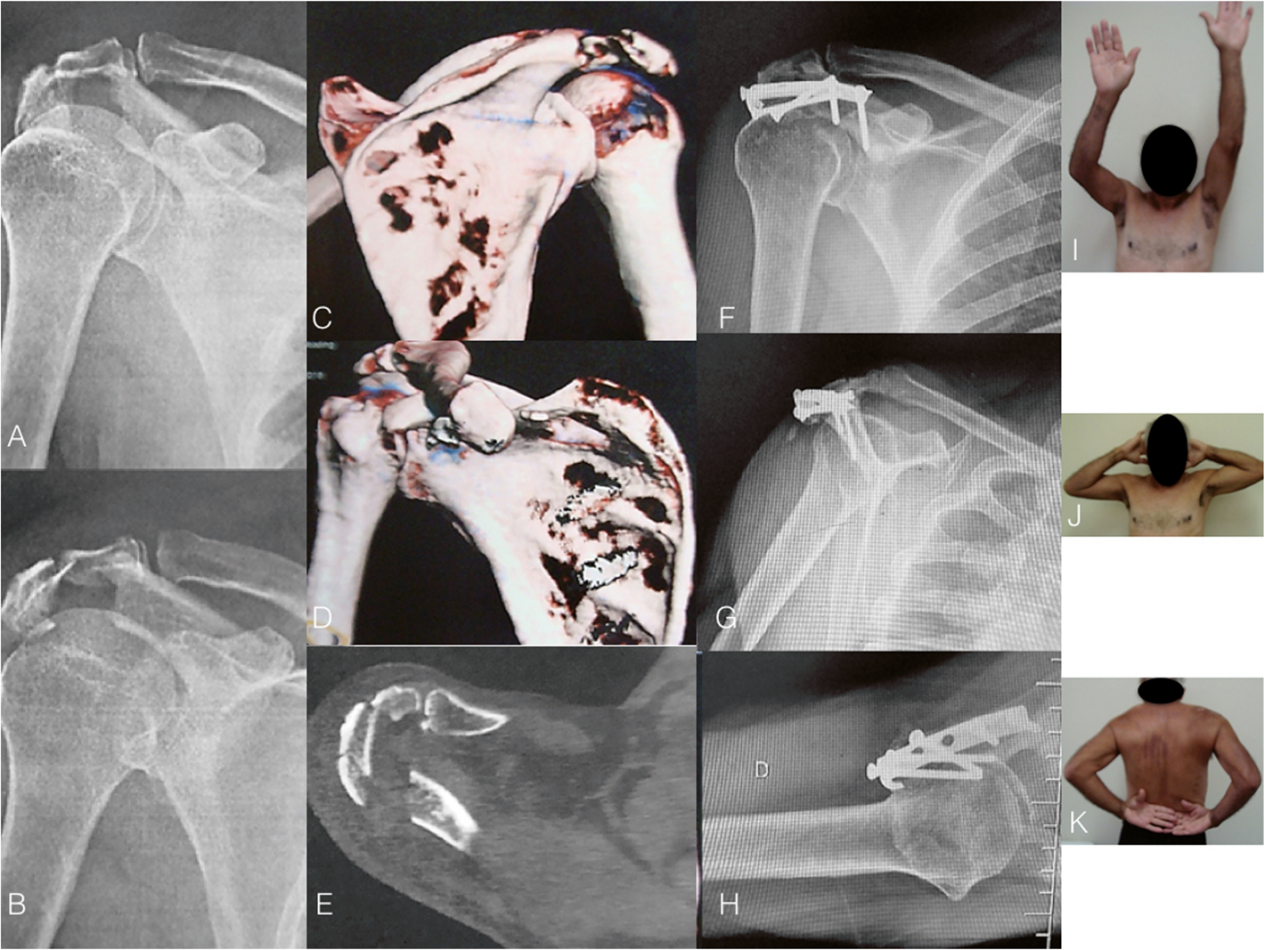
a and b: Radiographs of the shoulder showing a Kuhn et al. [18] type-II multifragmentary fracture of the acromion extending to the most lateral part of the scapular spine. c, d, and e: Observe the amount of comminution on the CT scan. There is no obvious reduction of the subacromial space. f, g, and h: Fracture fixation was performed with a superiorly placed non-locked one-third tubular plate. i, j, and k: Observe the functional range of motion of the operated shoulder after fracture healing at 24 months postoperatively
